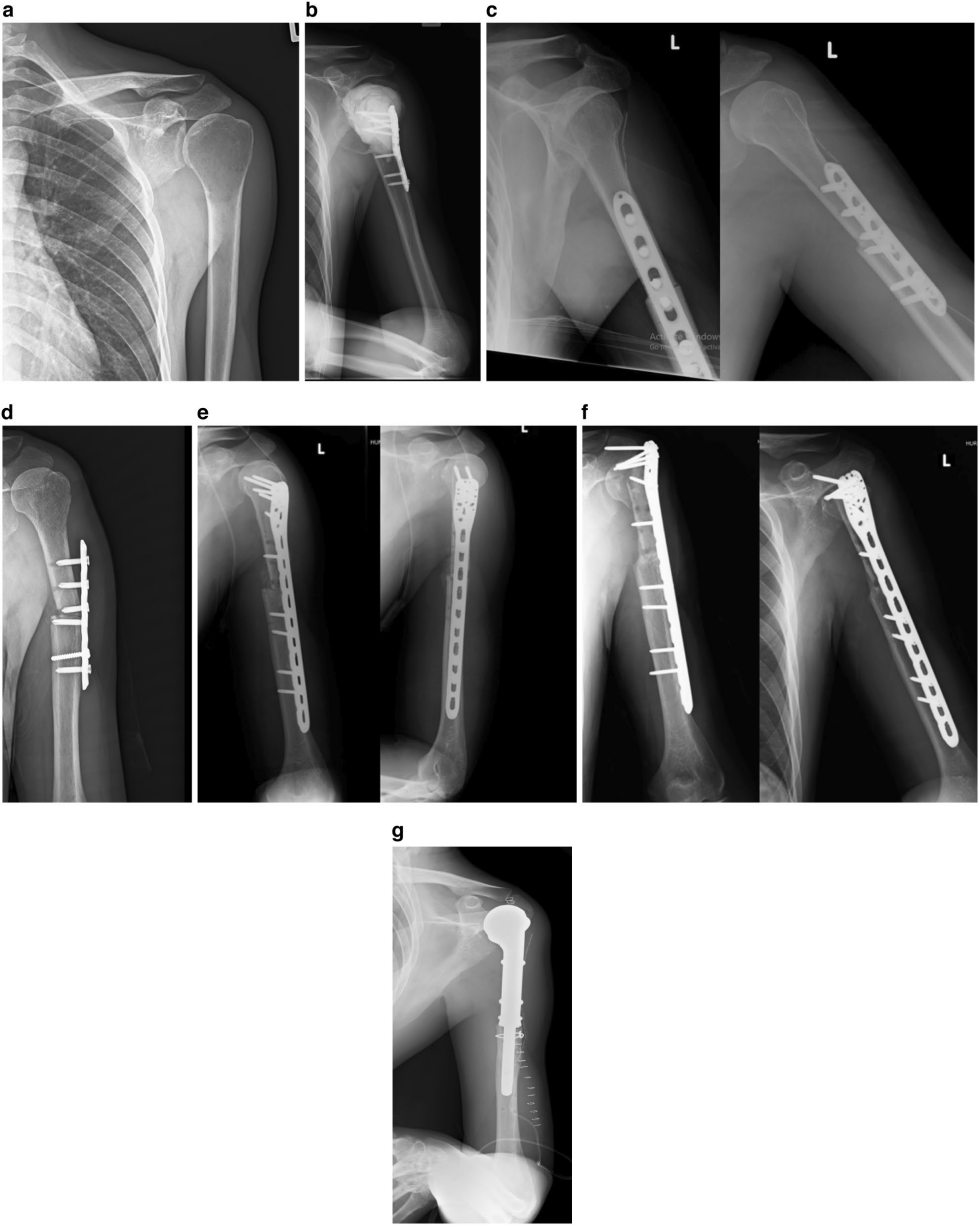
A 31-year-old male with giant cell tumor of the proximal humerus. (a) Pre-operative anteroposterior radiograph. (b) Six months after extended curettage with phenol and bone cement, tumor recurrence was detected. (c) Wide excision and allograft reconstruction was performed. (d) Three months later, failure of the implant was found. (e) Revision surgery with locking plate and screws was done. (f) Fifteen months after the revision procedure, fracture and lysis of the allograft occurred. (g) Finally, proximal humeral endoprosthesis was performed.
